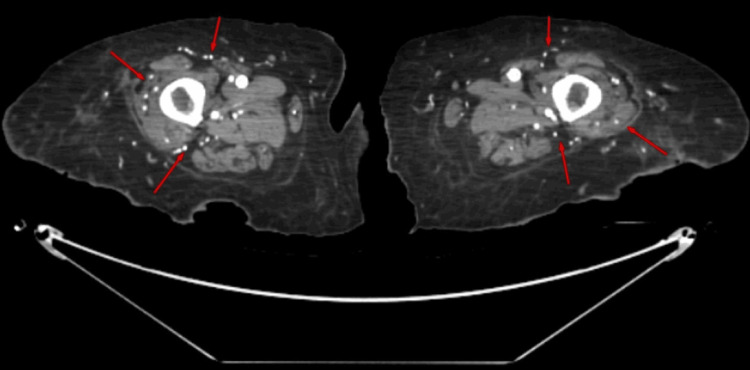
Computed tomography of the lower extremities showed extensive arterial calcifications.
